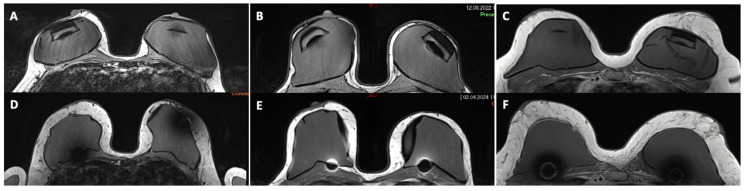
MRI examinations of a 37-year-old (A), 44-year-old (B), and 46-year-old (C) patient after a bilateral mastectomy sparing both skin and nipple–areolar complexes and pre-pectoral insertion of a breast tissue expander, before repeated sessions of autologous fat grafting of the mastectomy flap and reverse expansion of the expander. In (D–F), MRI examinations performed 3 months after 3 sessions of autologous fat grafting each and exchange of the expander with a definitive implant. Note the mean gain of tissue thickness in both breasts of 320% (D), 240% (E), and 120% (F).
